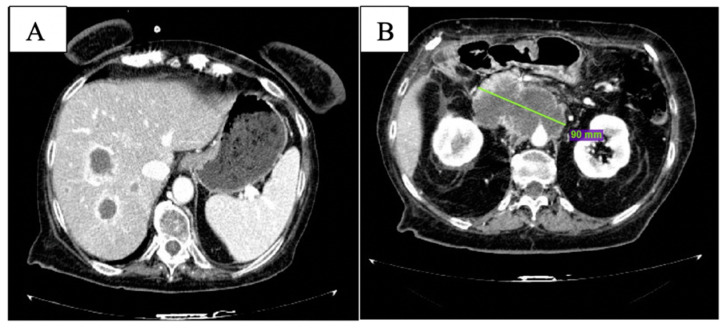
CT scan after 6 months of olaparib: new liver lesions are documented (A) and peripancreatic tissue is increased (B).
